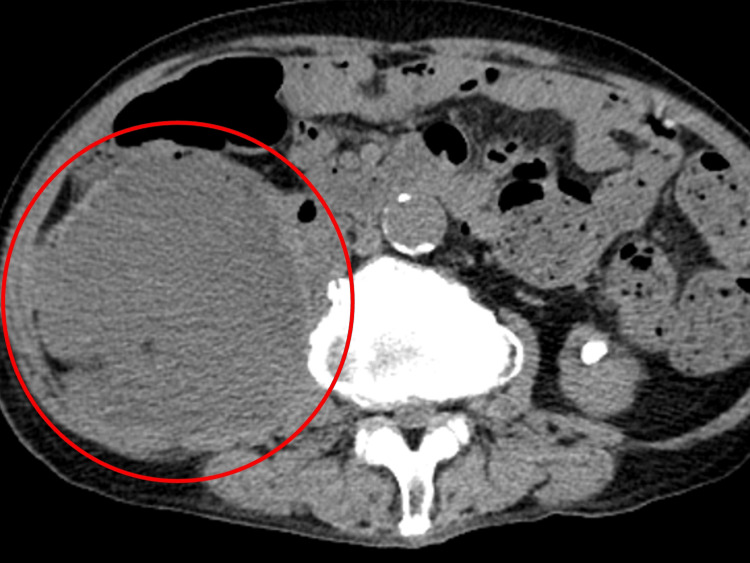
Abdominal CT revealing a well-defined, heterogeneous right retroperitoneal mass containing intralesional gas (red circle).
